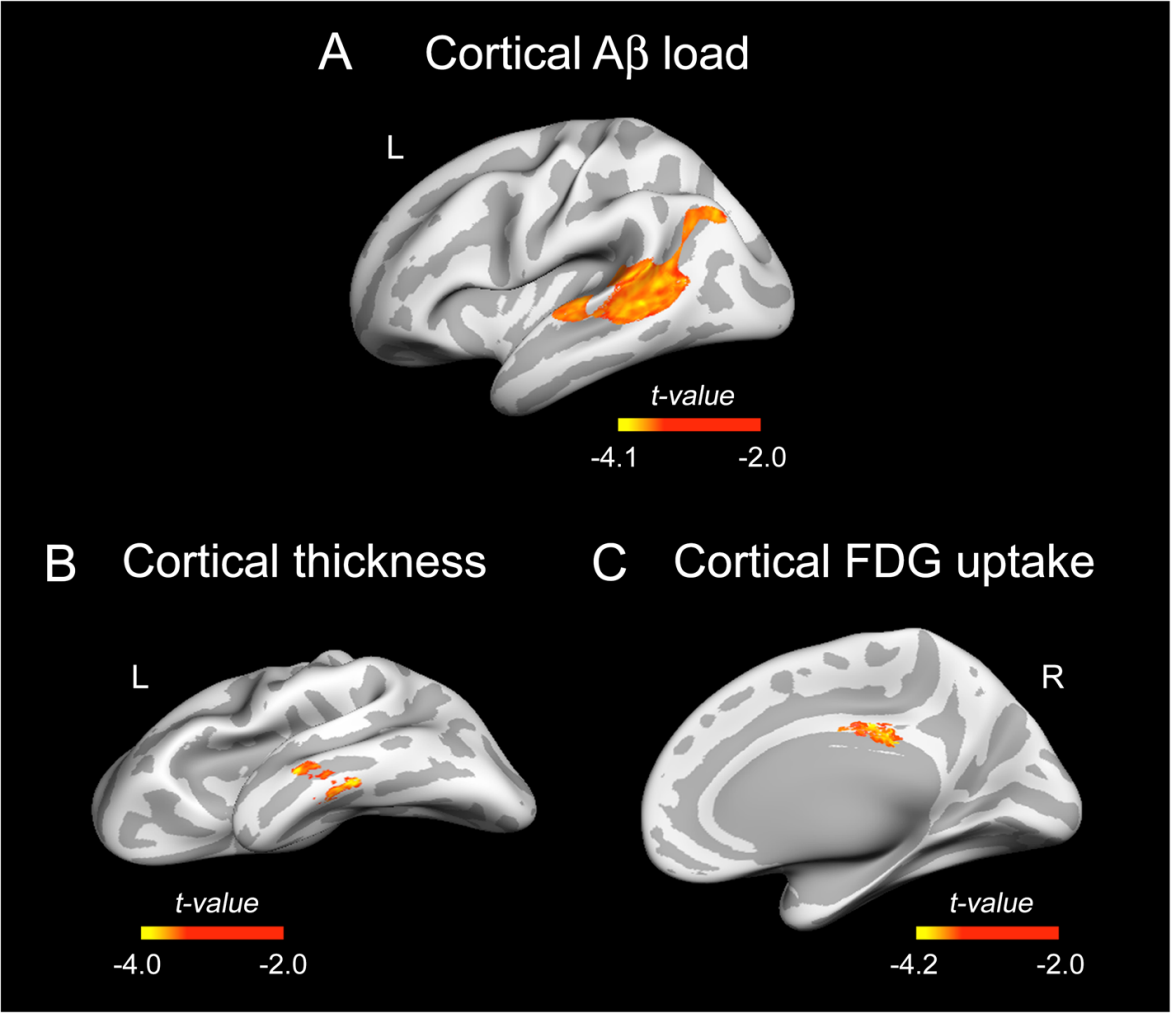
Association of sLF with cortical Aβ load, cortical thickness and cortical FDG uptake. The *t* statistics projected into the inflated cortical surfaces indicate the negative associations of sLF with cortical Aβ load (A) after adjustment for age and sex and with cortical thickness (B) and cortical FDG uptake (C) after adjustment for age, sex, and global Aβ load. All cortical measurements were Box-Cox transformed before whole-cortex vertex-wise analysis. Color bar indicates the range of significant *t* values. Left (L) and right (R)
